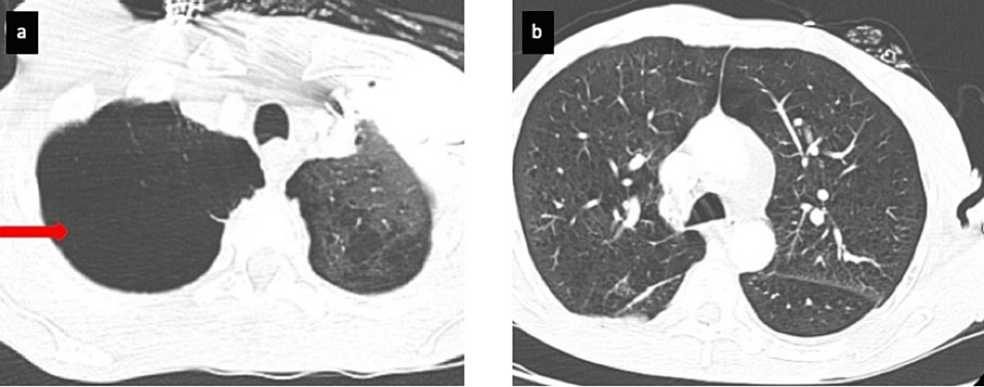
Lung window of computed tomography pulmonary angiography (CTPA) scan showing (a) large right apical lung bulla (red arrow) and (b) emphysematous changes in both lungs
